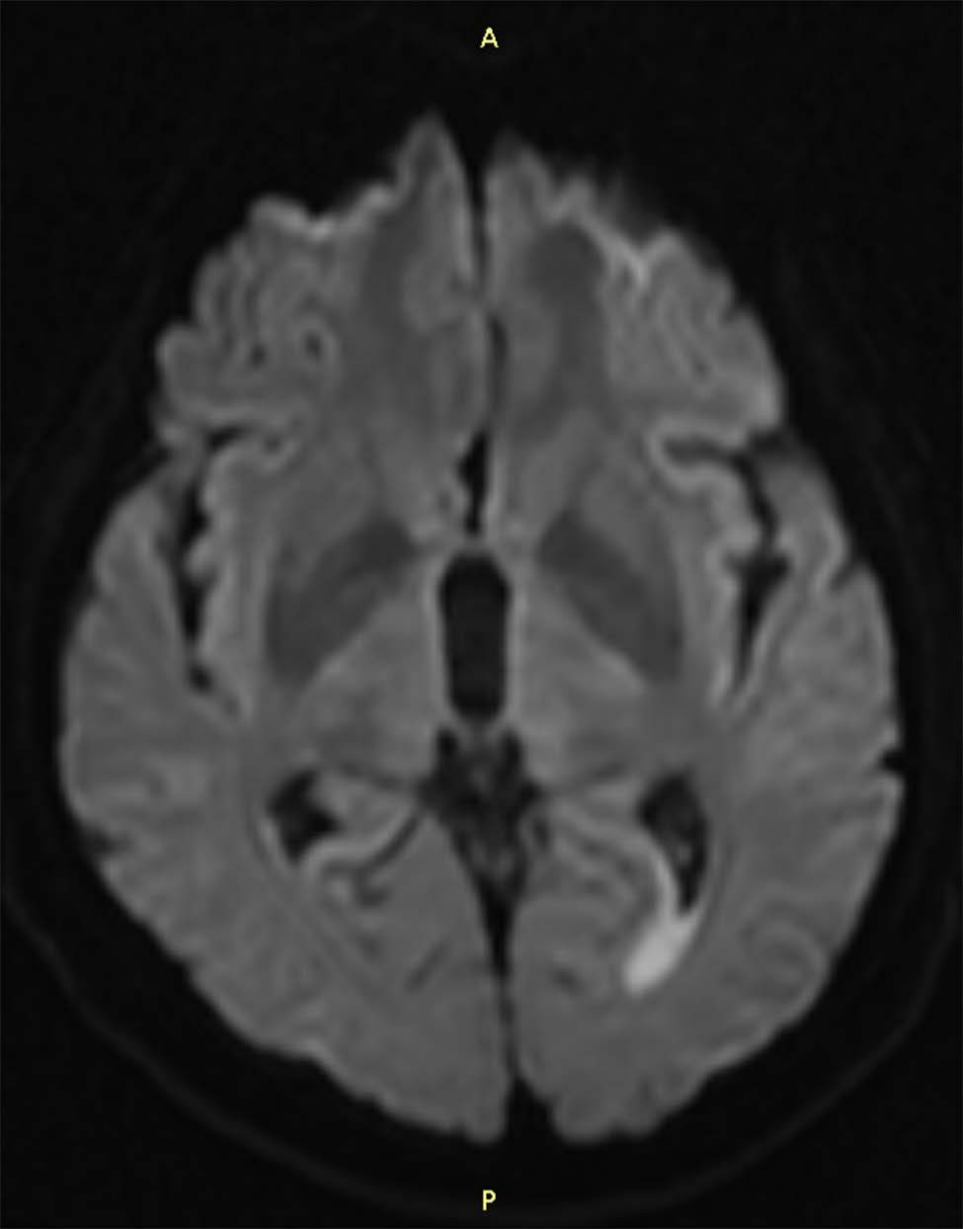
The lesion of the posterior horn of the newly developed lateral ventricle in DWI b1000 (September 14, 2022). A line segment is drawn from the farthest end of the posterior corner of the left lateral ventricle toward the farthest end of the lesion, with a length of 1.56 cm. The angle between the segment and the horizontal line is 60°. The maximum width of the lesion is 0.78 cm, and the maximum circumference is 7.98 cm. The signal of the lesion is high, with an average intensity of 319. The number of visible layers of the lesion is 2 (slice thickness = 5 mm).
